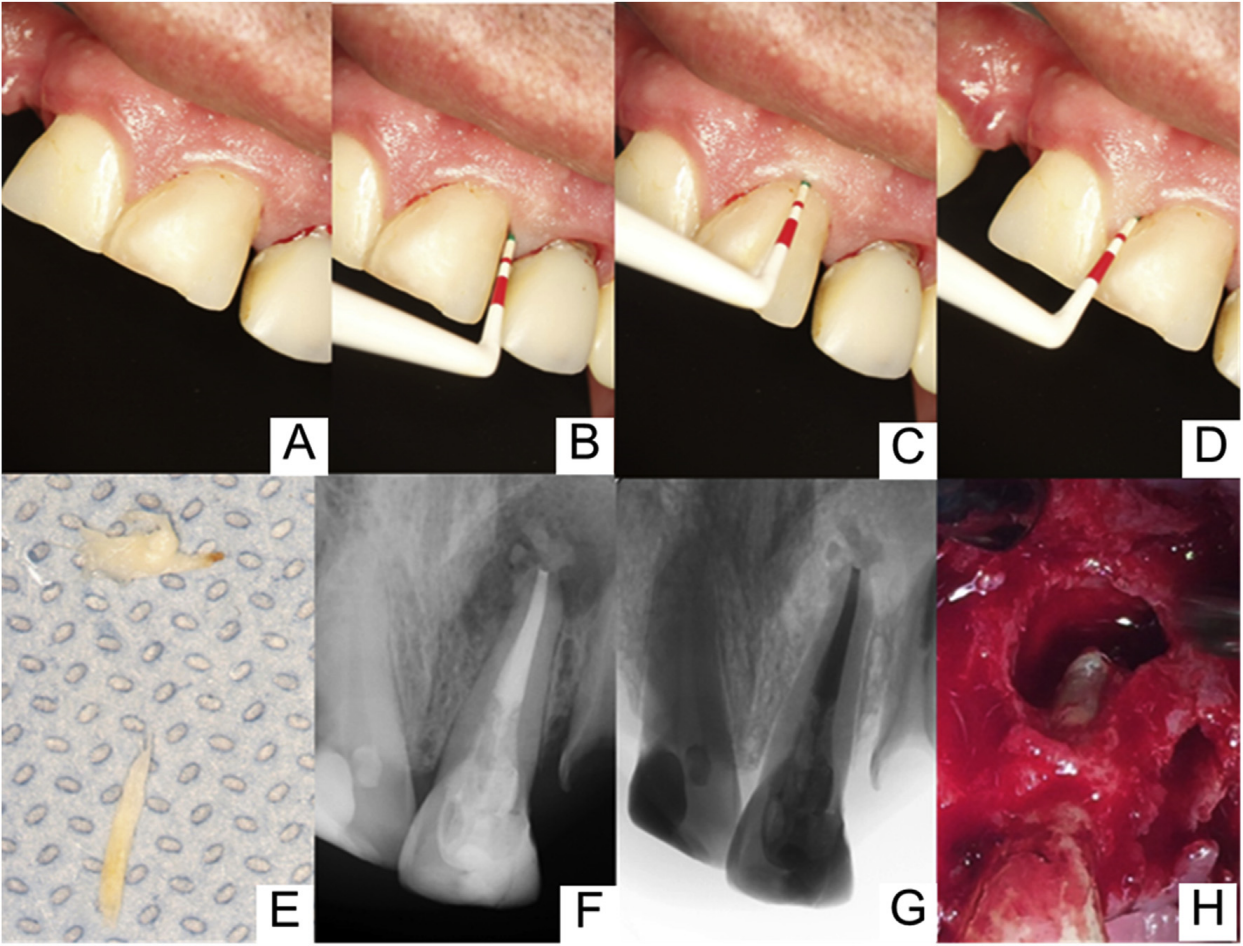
(A–D) Maxillary left central incisor showed no significant periodontal probing depth; (E) Fragments of cotton ball and cone of absorbent paper removed from canal; (F–G) Radiographic exam of final canal filled; (H) Surgical access.
